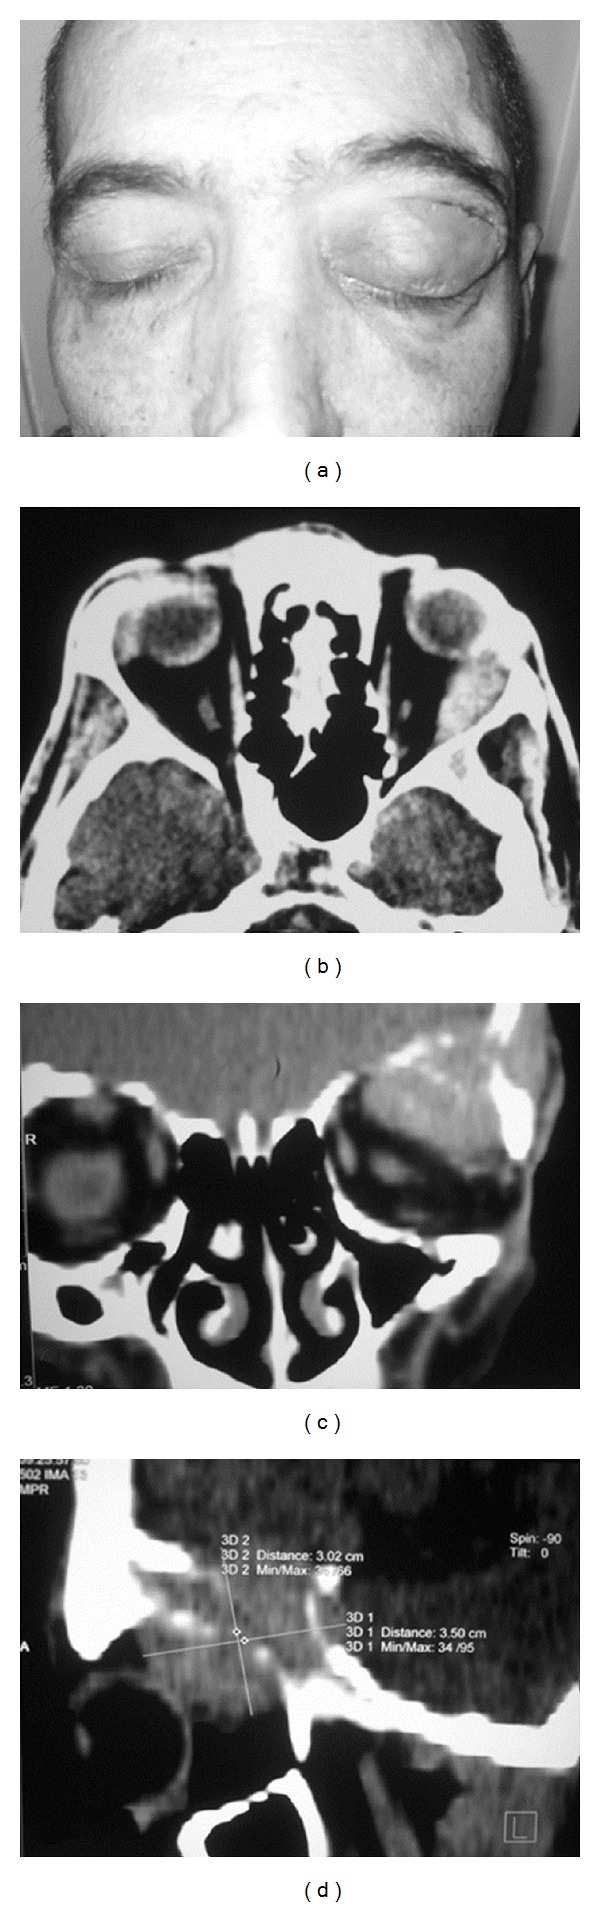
(a) Proptosis of the left eye, (b), (c), and (d) computed tomography (CT) scans of the orbit that showed an extraconal mass involving the superior and lateral wall of the left orbit with destruction of the orbital bone, incorporating the lateral rectus, with calcifications.
